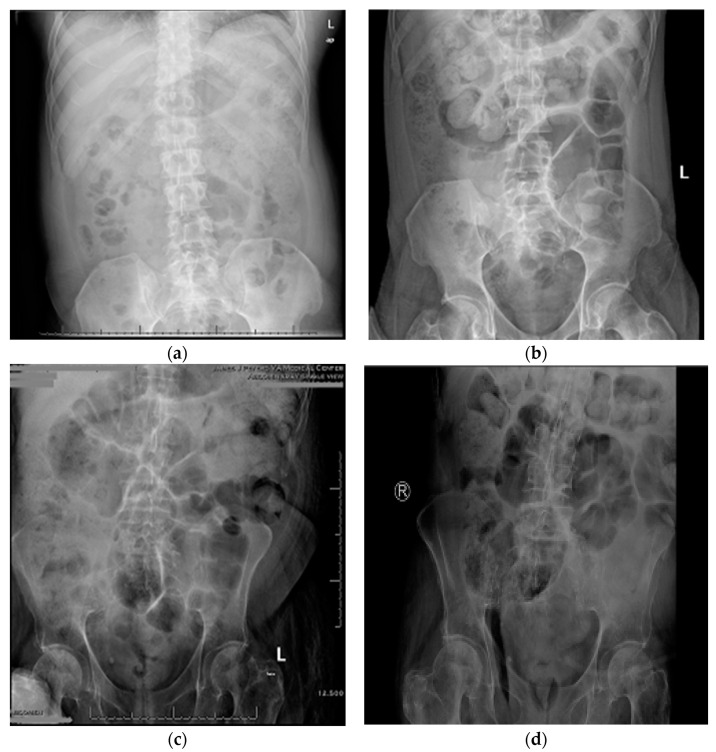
Representative qualitative measure of stool burden on abdominal radiograph after standard of care or standard of care plus neostigmine and glypyrrolate. Fecal burden: (a) marked stool throughout the colon, (b) moderate stool in the cecum; (c) moderate stool in the transverse and left colon, (d) moderate stool in the cecum. Loss of body weight after two weeks of standard of bowel care and the dual drug combination: (a,b), −4.4 kg; (c,d), −2.6 kg.
